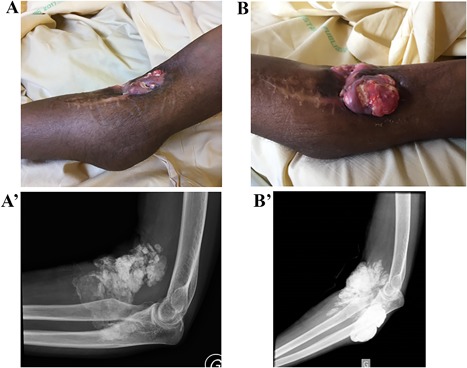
Calcifications surrounding the left elbow. Clinical aspect (with ulcer and visibility of the calcification) and radiographs showing the changes between December 2017 (*A, A’*) and March 2018 (*B, B’*).
